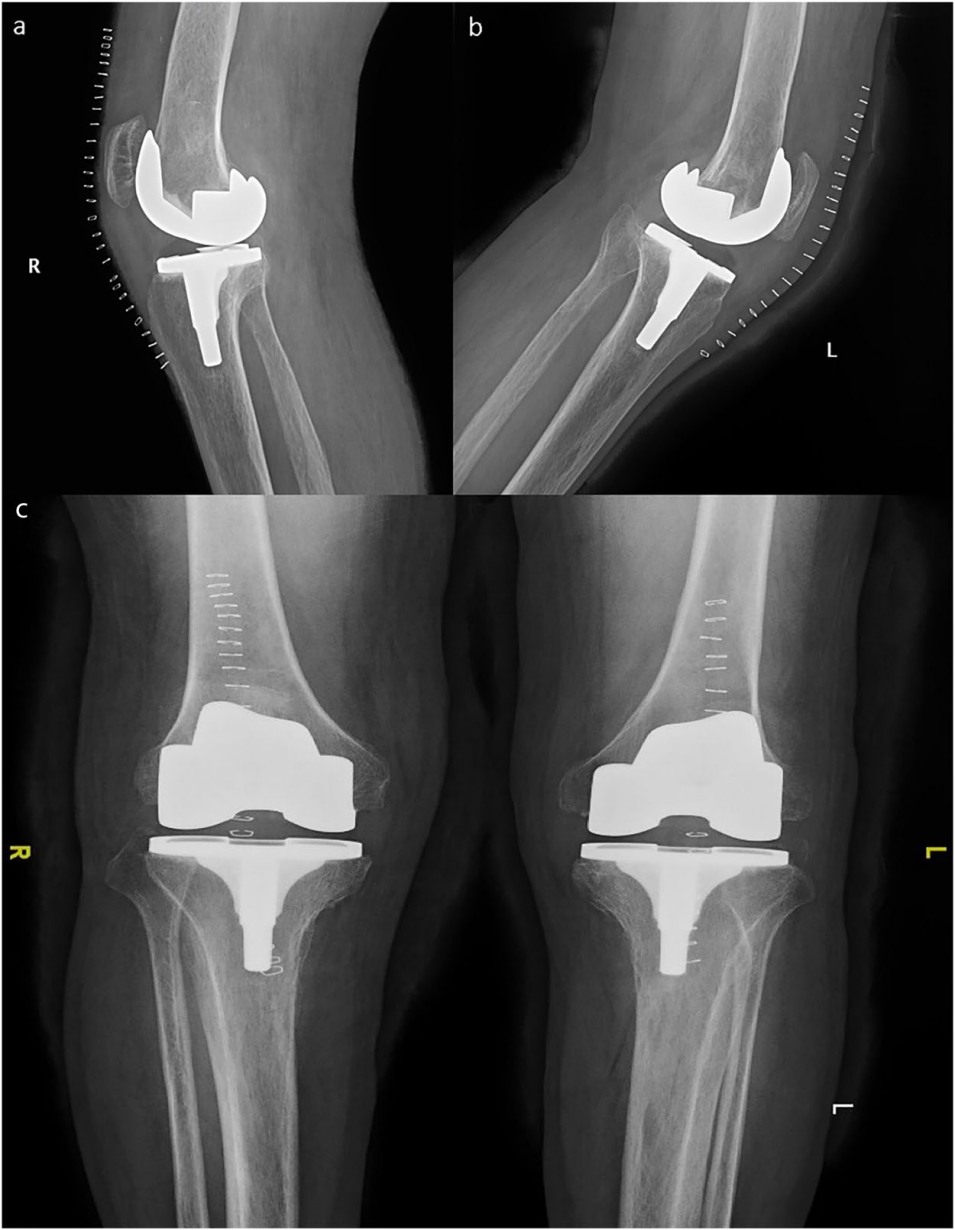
Postoperative X-ray image of the same patient presented in Fig. 1. (a) Lateral view of the right knee joint. (b) Lateral view of the left knee joint. (c) Lnteroposterior view of both knee joints.
